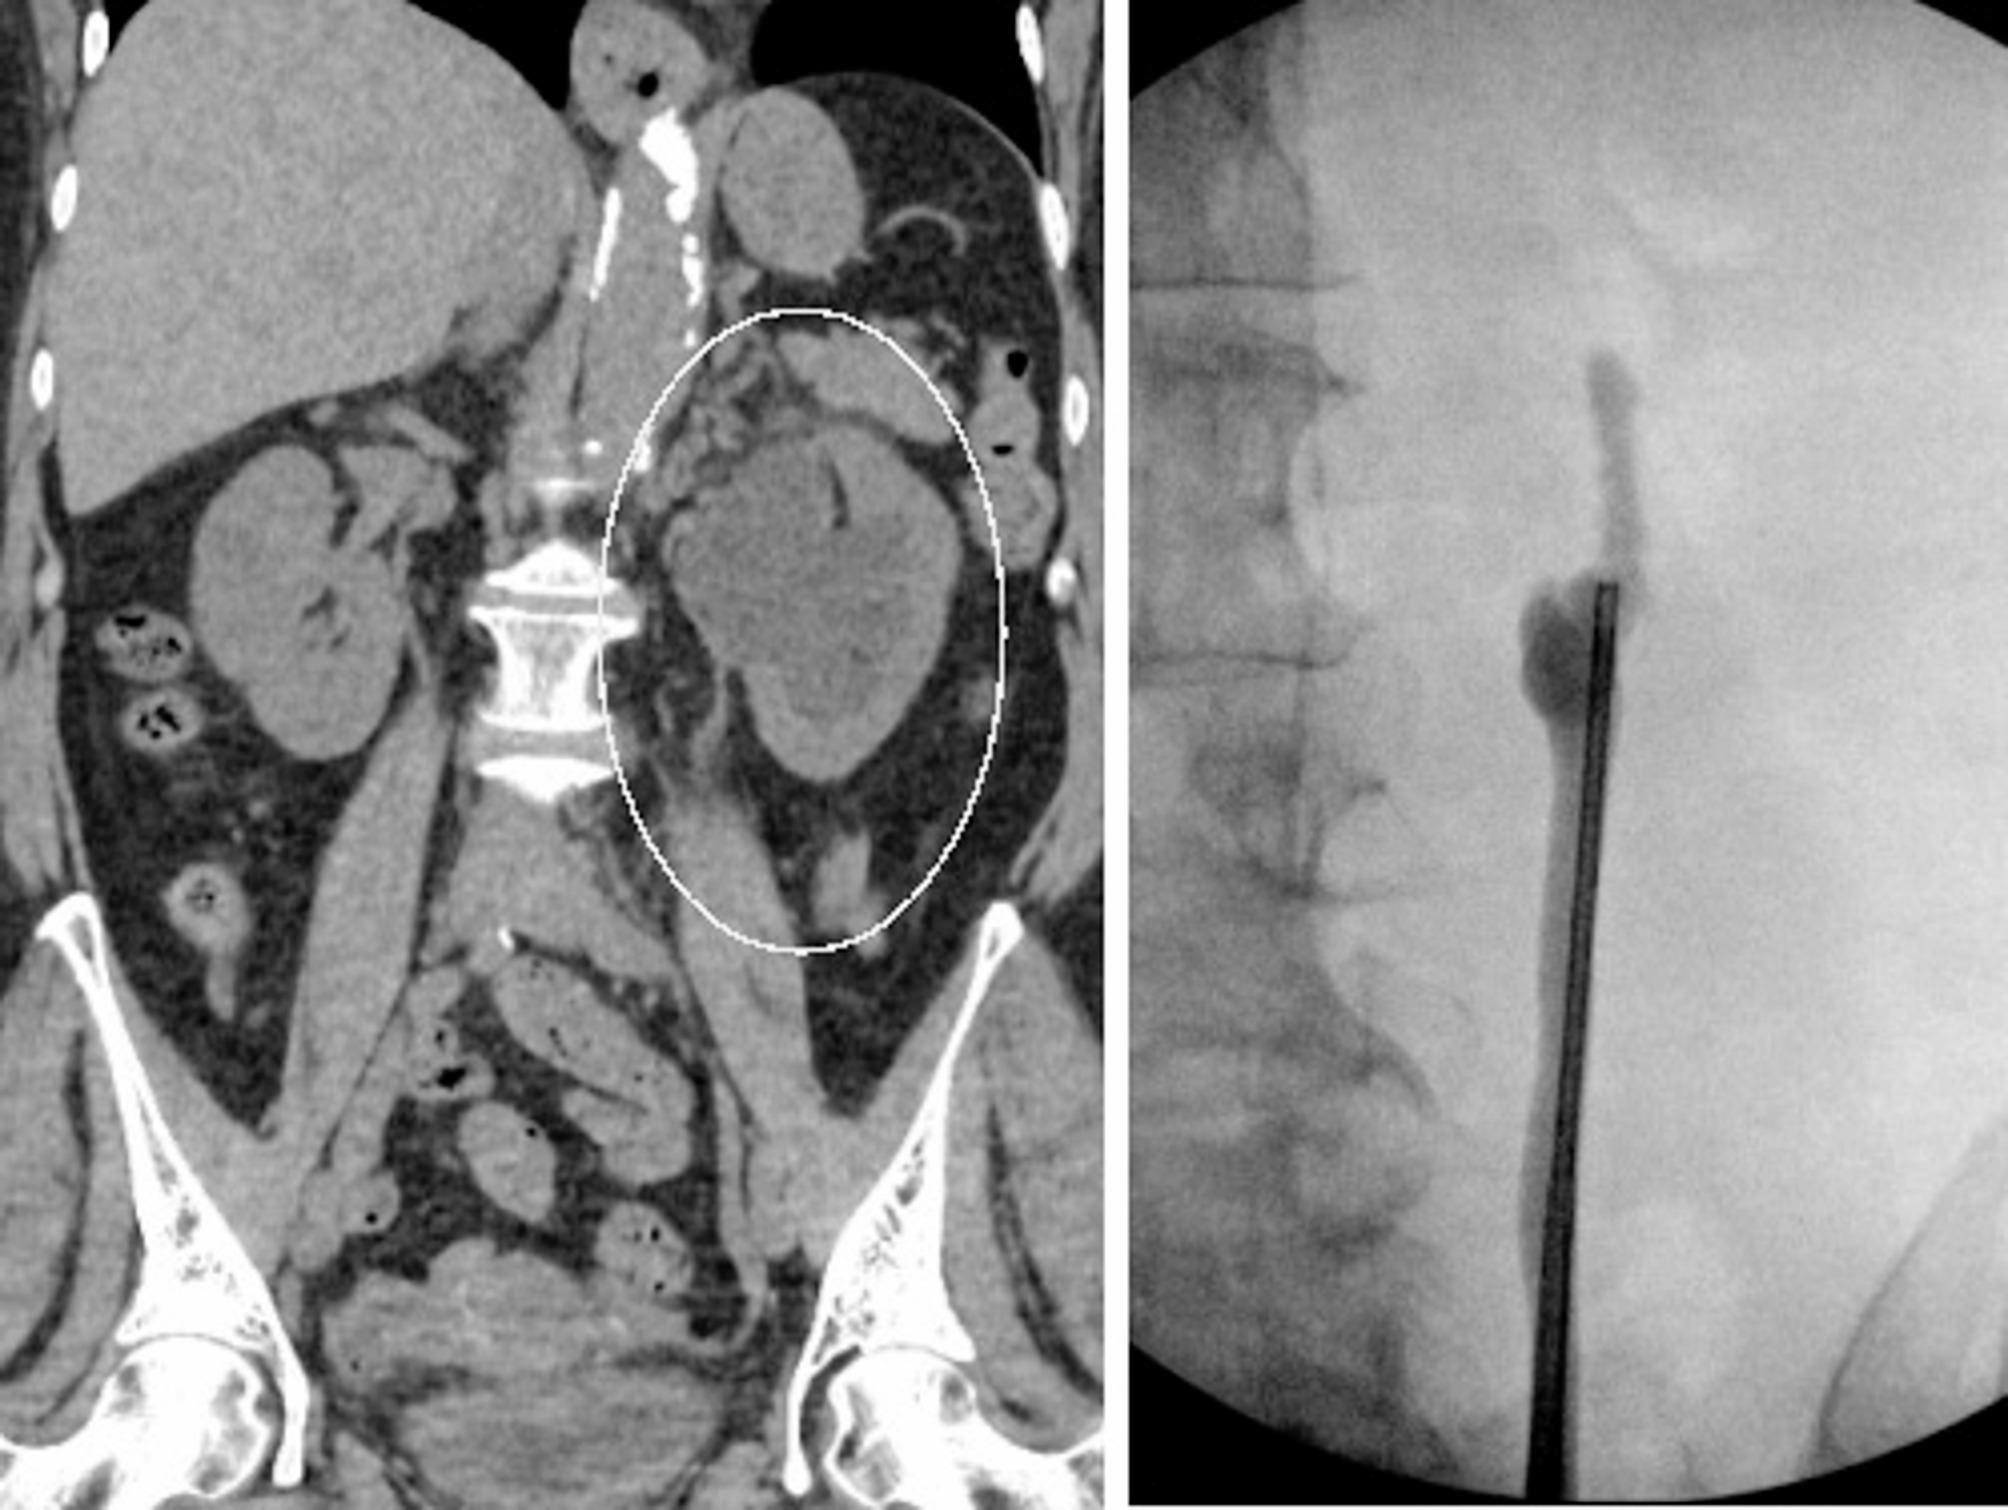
Representative computed tomography (CT) image of a patient and their associated retrograde fluoroscopic imaging study demonstrating an obliterated stricture
